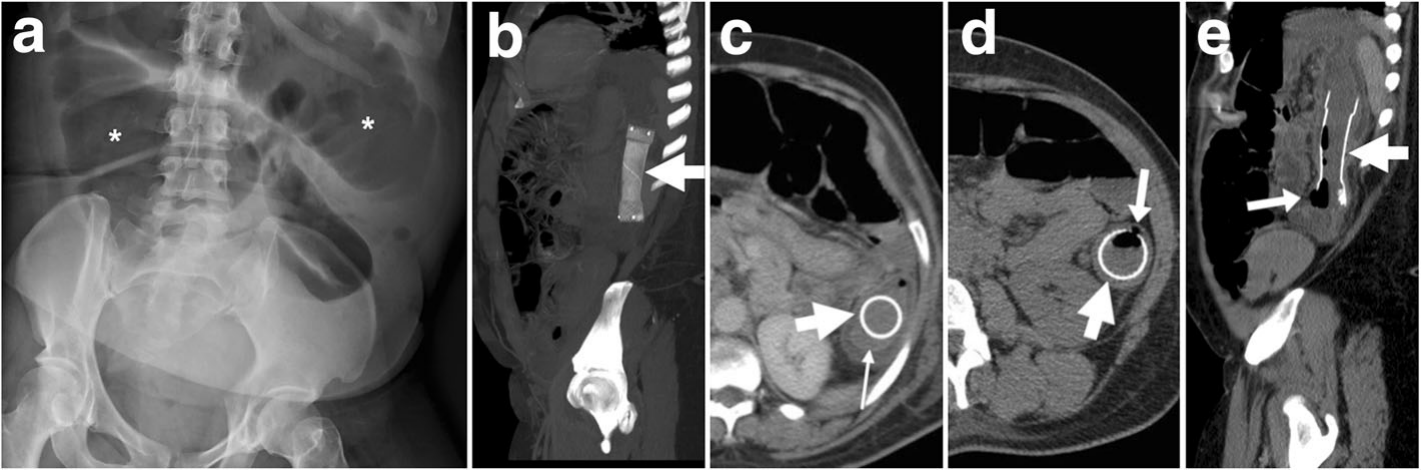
Haemorrhage and impending stent-related perforation. Initial radiograph (a) showing marked colonic dilatation (*). After early clinical improvement, the patient experienced haematochezia. MIP CT image (b) confirmed correctly placed and expanded SEMS (thick arrows) at the descending colon. CT images showed some hyperattenuating fresh blood (thin arrow in c) in the bowel lumen and a gas bubble (arrows in d and e) piercing through the colonic wall at the distal end of the SEMS. Emergency laparotomic surgery (left hemicolectomy with primary anastomosis) was required
